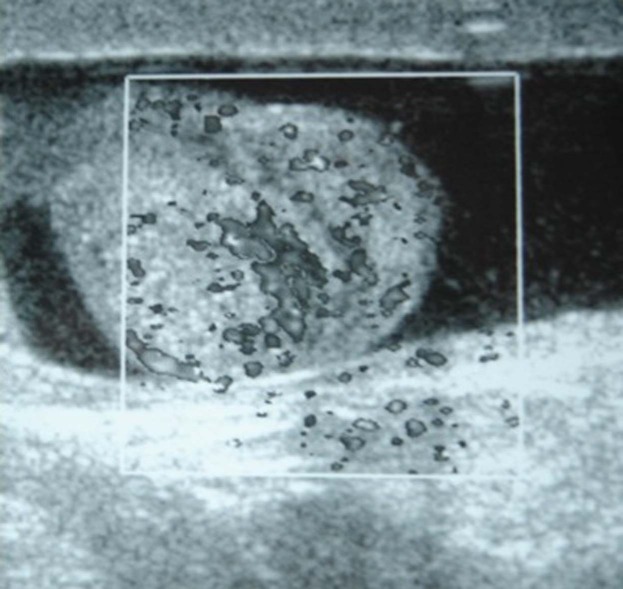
Color Doppler Ultrasonography Image Shows Increased Vascularization of the Left Epididymis.
